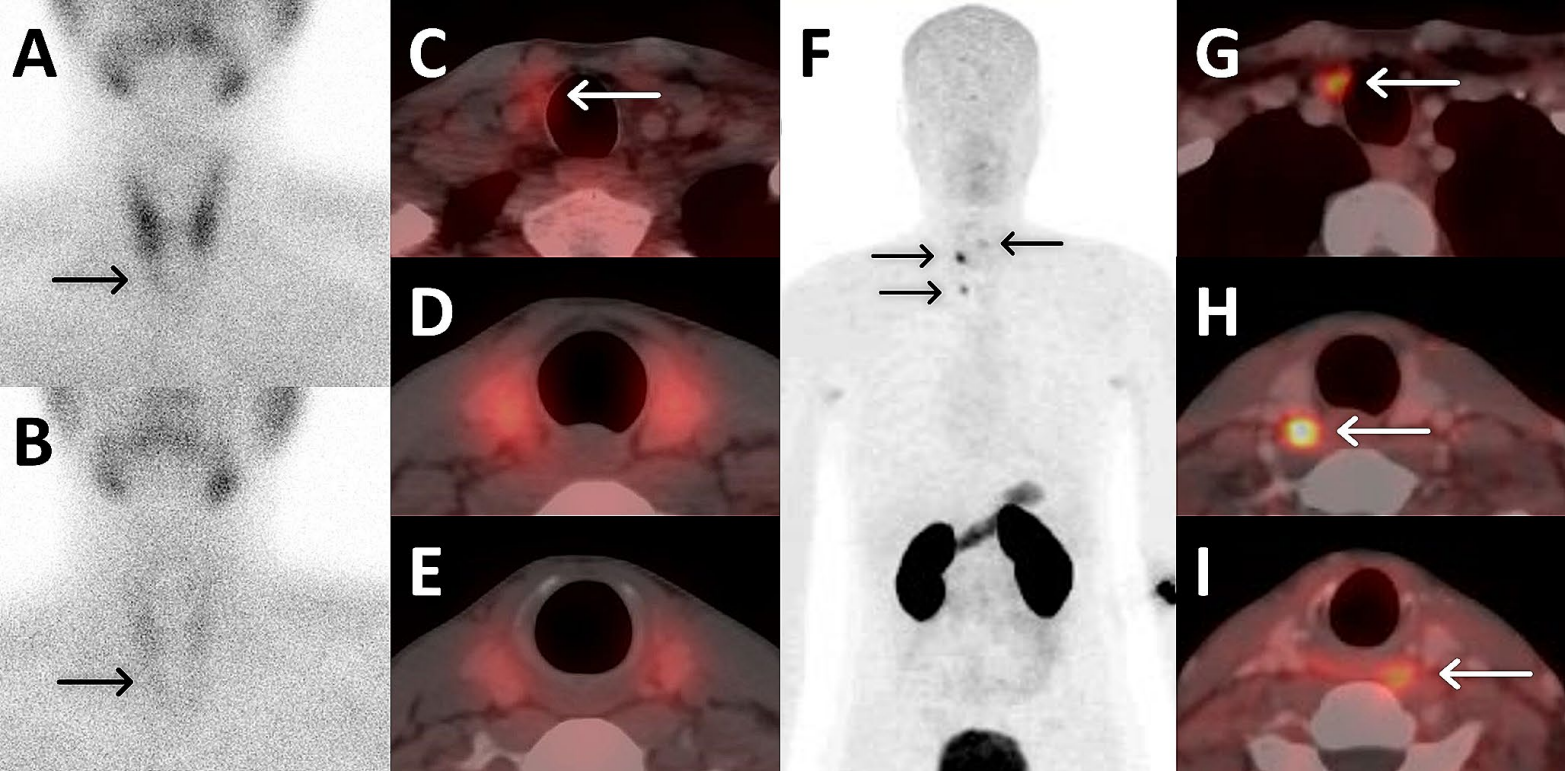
A 38-year-old man with MEN-1 syndrome and associated PHPT (PTH: 110 ng/l, Calcium: 11.4 mg/dL) underwent MIBI scan, revealing uptake in a lesion inferior to the right lobe of the thyroid gland on planar (A, B) and SPECT/CT (C) images. However, no accumulation was detected in lesions located posterior to the right lobe and in the left upper lobe (D, E). Subsequent ^68^Ga-Trivehexin PET/CT revealed uptake in all three lesions (F, G, H, I)
